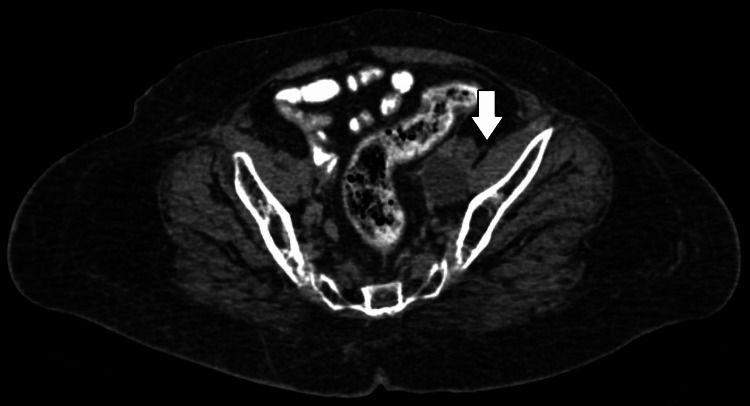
Response CT showing further reduction of lymphocele CT: computed tomography
